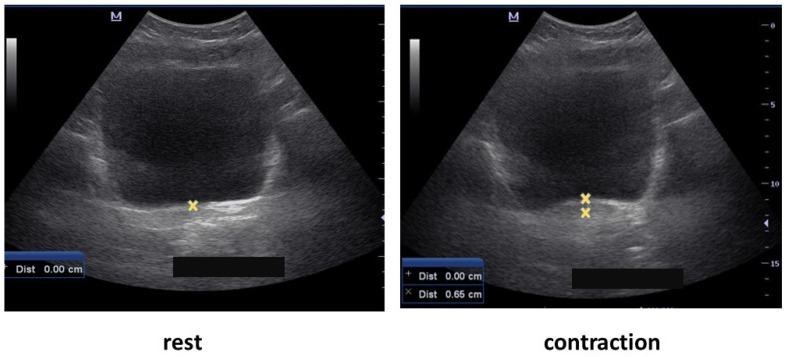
Ultrasound assessment of pelvic floor muscle function: Displacement during rest and contraction.
